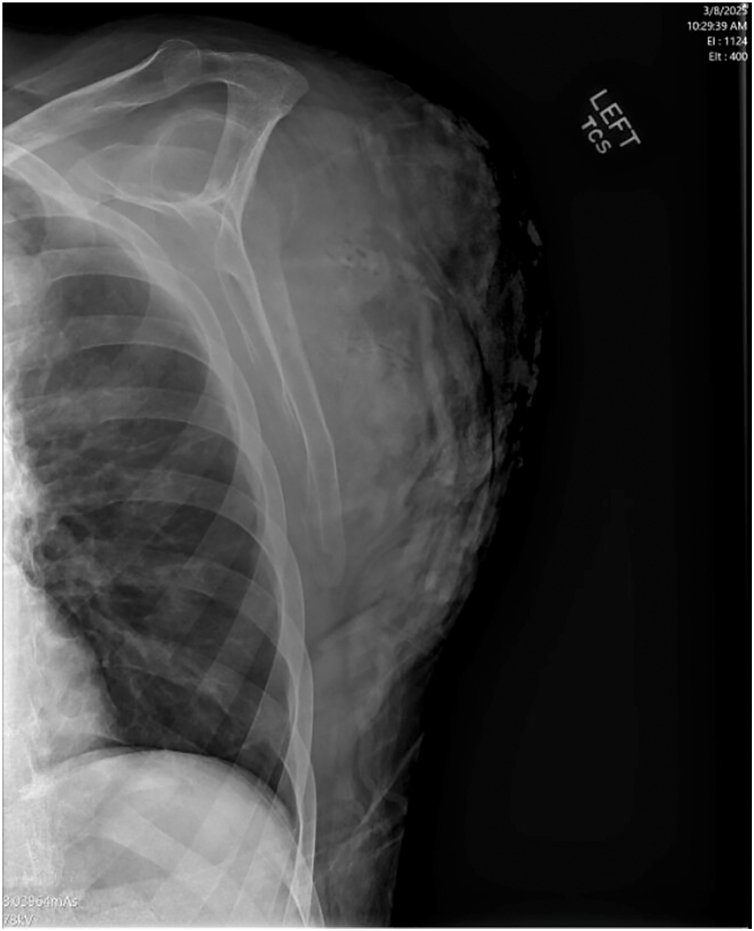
Post-operative scapular Y radiograph of the left shoulder.
